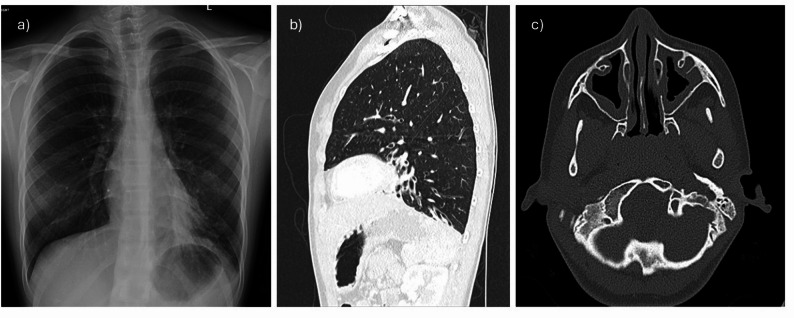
Clinical radiology studies on the female patient with X-linked agammaglobulinemia. a Posterior-anterior chest radiography with left lower lobe opacity at presentation, b Sagittal view of chest computed tomography showing cylindrical bronchiectasis in the lingula and left lower lobe, c Computed tomography of the head showing bilateral mucosal thickening of the maxillary sinuses
